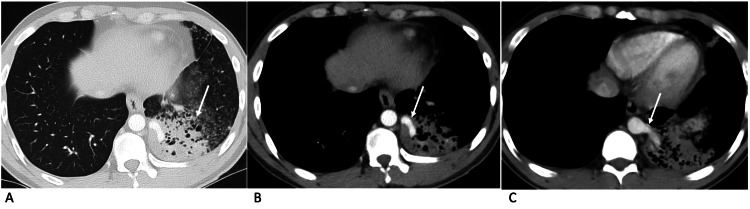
Case 1 - axial images of contrast-enhanced CT of the thorax Axial images of contrast-enhanced CT of the thorax in lung window (A) and mediastinal window (B and C) demonstrate pulmonary sequestration in the left lower lobe (arrow in A) supplied by an arterial branch arising directly from the descending thoracic aorta (arrows in B and C).
